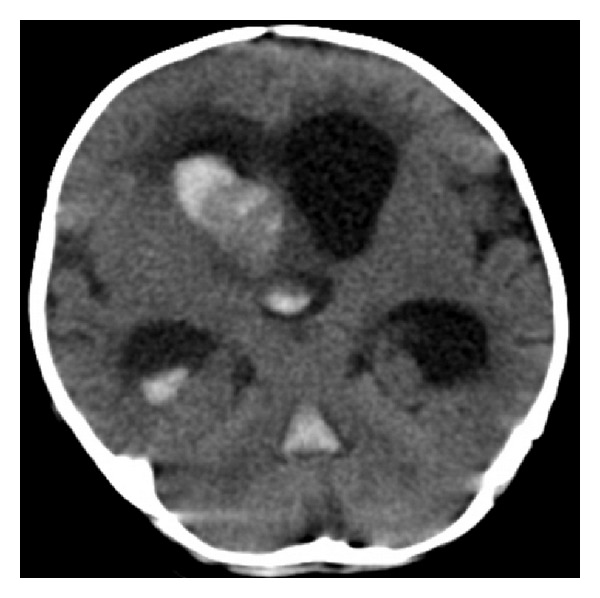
Cranial preoperative CT-scan: tetraventricular hemorrhage (Fisher 4) and acute hydrocephalus.
